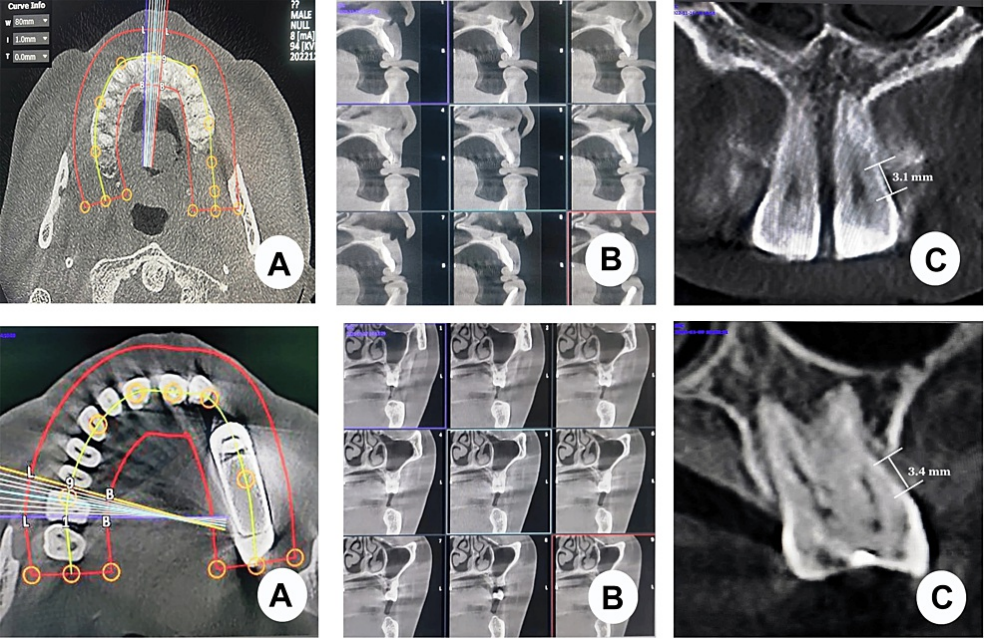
CBCT assessment of periodontal bone loss in anterior teeth (upper A, B, and C) and posterior teeth (lower A, B, and C). CBCT, cone-beam computed tomography
